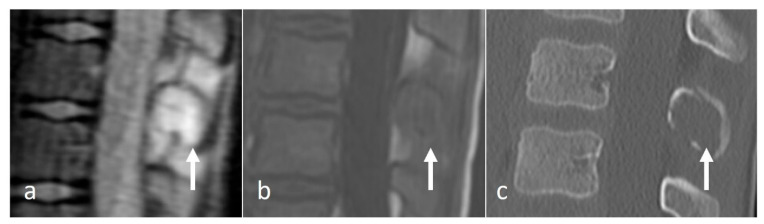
Sagittal STIR (a), T1 (b) and CT (c) images demonstrating an eosinophilic granuloma of a thoracic spinous process (white arrows) in a paediatric patient. The lytic appearance is typical of these lesions.
